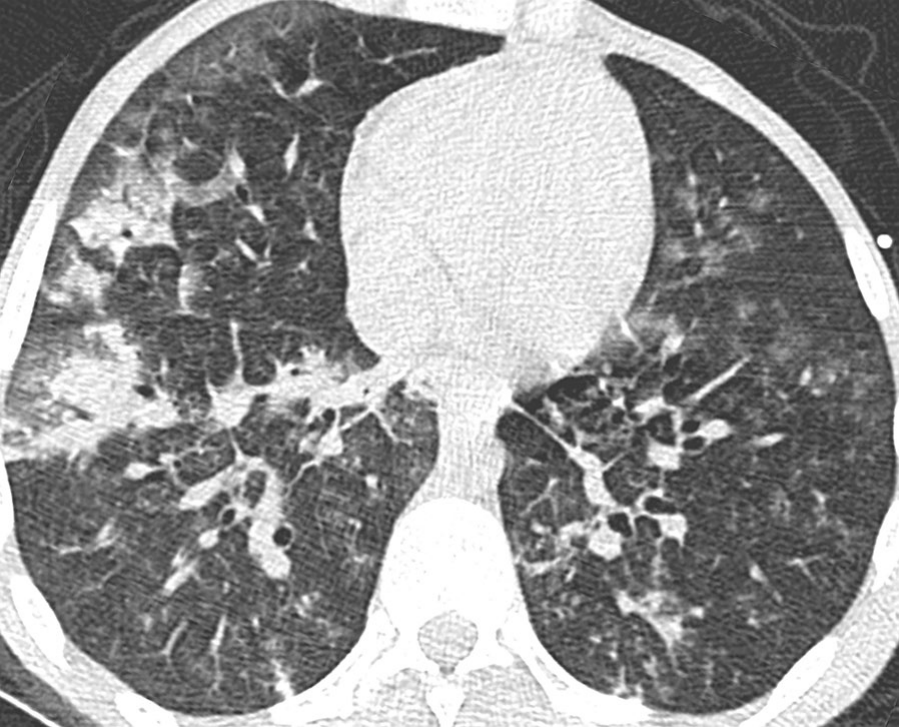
Bacterial super-infection in COVID-19 pneumonia. CT scan was obtained in a patient previously diagnosed with COVID-19 pneumonia upon clinical deterioration. Axial CT image shows bilateral peribronchovascular nodular GGOs, centrilobular nodular opacities, and consolidations in the right lung. Peripherally located GGOs are observed in the right middle lobe. Respiratory tract sample was positive for Hemophilus influenza
